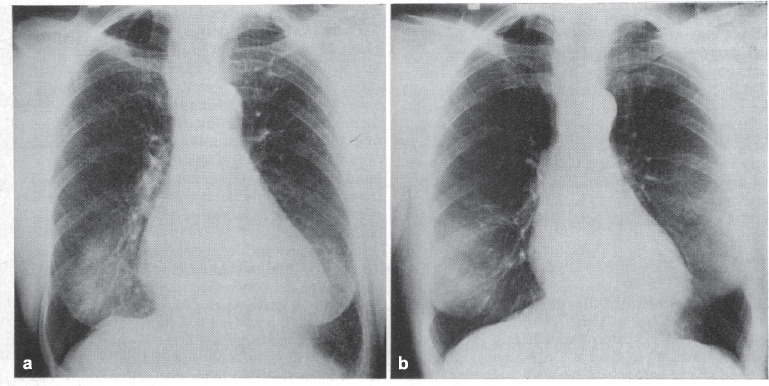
Chest X-ray of a female patient before (a) and after (b) 12 months of treatment with the unselective β-blocker alprenolol (50 mg twice daily) on top of digoxin, furosemide, and quinidine (for atrial fibrillation). Reproduced from (Waagstein et al. 1975), with permission of the publisher
